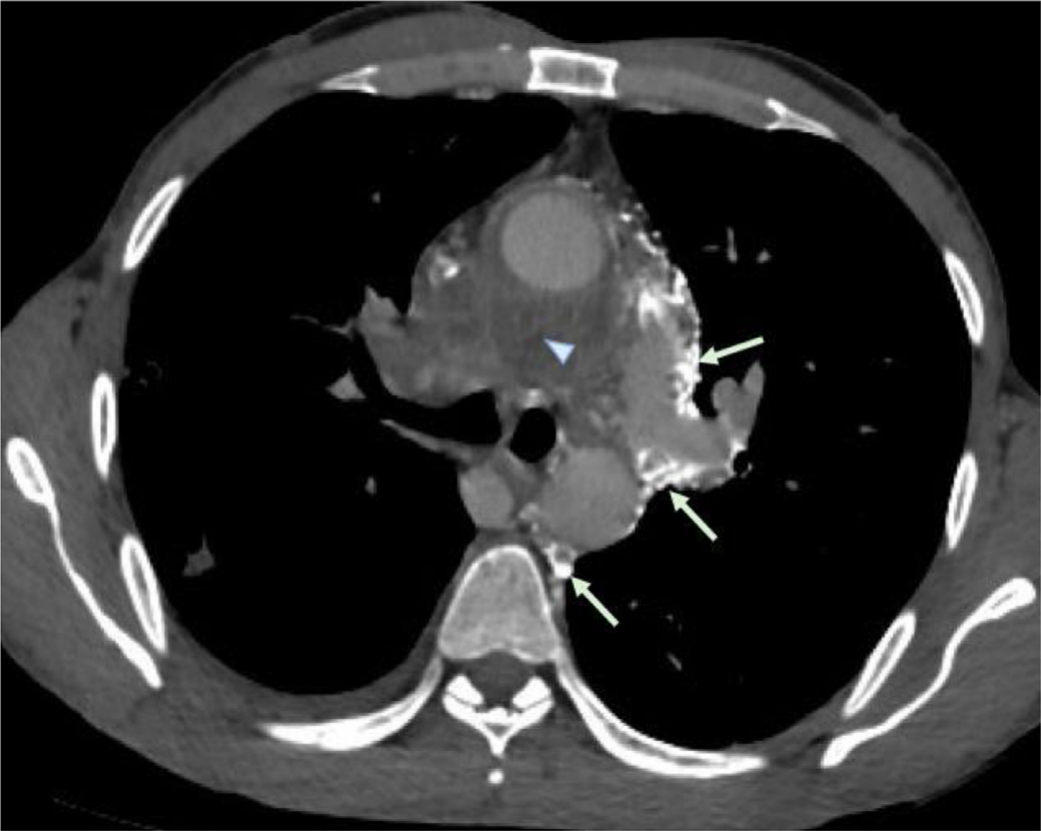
Axial contrast-enhanced CT image (soft-tissue window) showing the extension of the soft tissue into the aorto-pulmonary window, the pre- and sub-carinal spaces, and also in the right hilar region (arrowhead). We note also multiple collateral channels draining into the hemi-azygos vein which is early opacified (Arrows).
